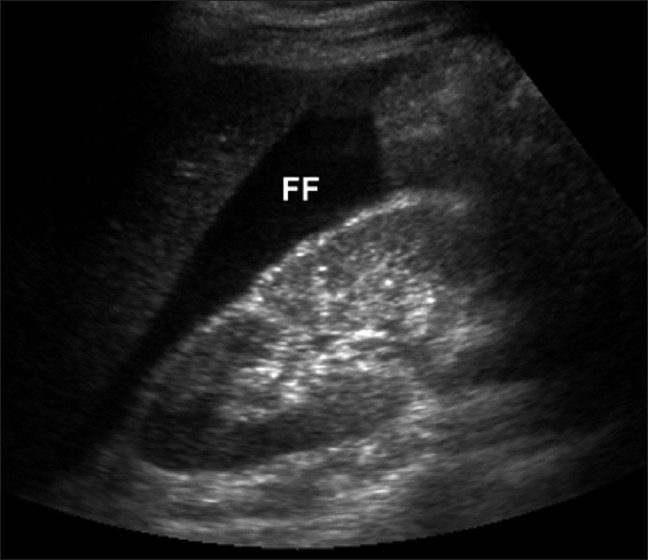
Ultrasonography demonstrating free fluid adjacent to the kidney, consistent with a large amount of hemoperitoneum in a patient with ruptured ectopic pregnancy
